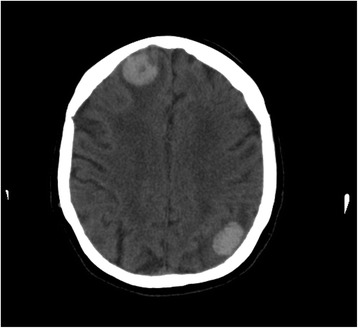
Computed tomography scan of the brain showed multiple areas of hemorrhage
